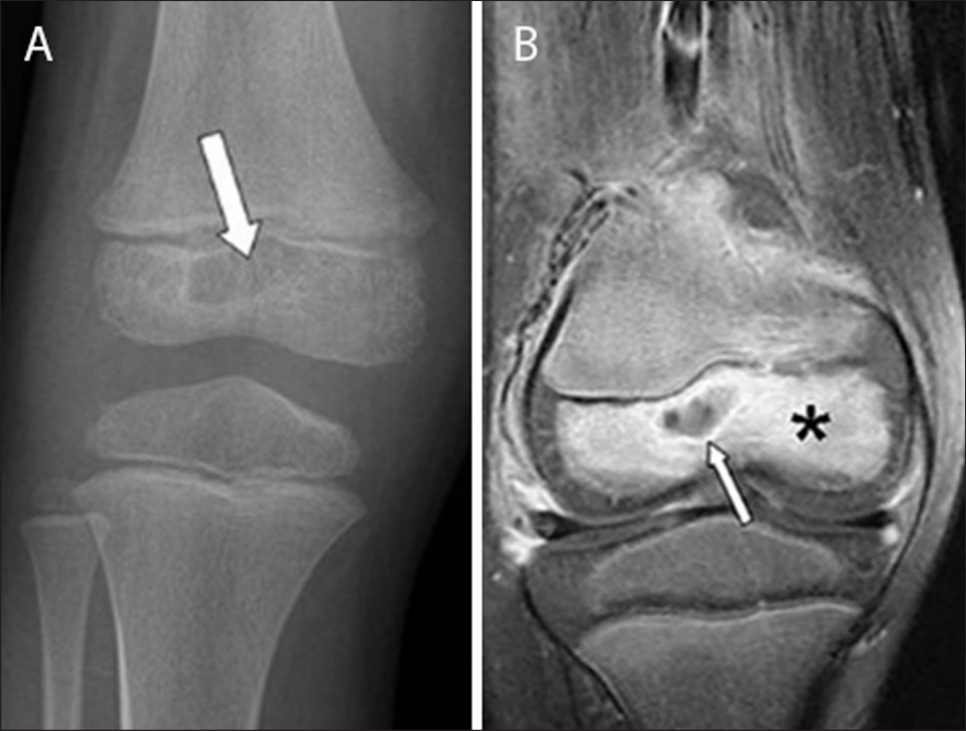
Epiphyseal childhood osteomyelitis of the right knee. Plain radiograph (a) of the distal femur shows a radiolucent lesion with peripheral sclerotic rim (white arrow) in the epiphysis of the distal femur. After gadolinium contrast administration (coronal T1–Fat–Sat WI, (b) the central part of the lesion is non-enhancing whereas there is subtle peripheral rim enhancement (white arrow) with moderate enhancement of the surrounding bone marrow edema.
